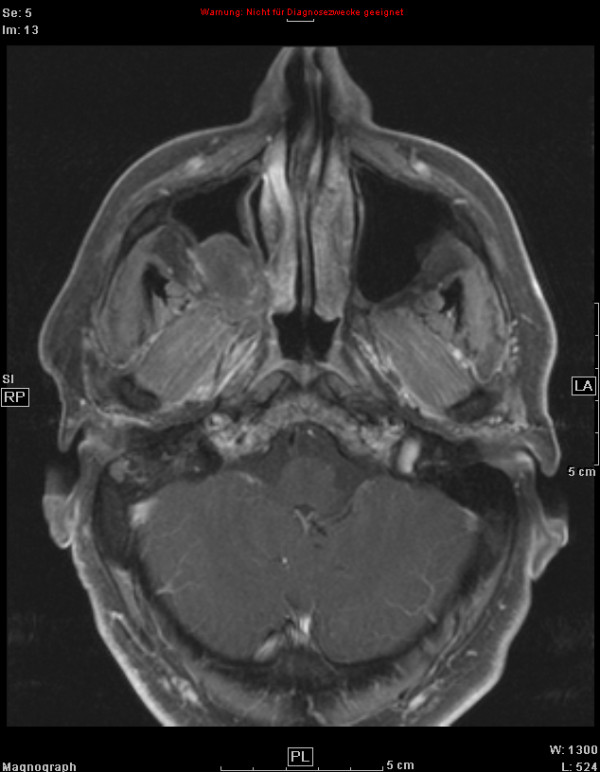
51 year old patient with adenoid cystic carcinoma, contrast-enhanced, T1-weighted MRI at first follow-up showing partial remission but highly reduced contrast-enhancement.
